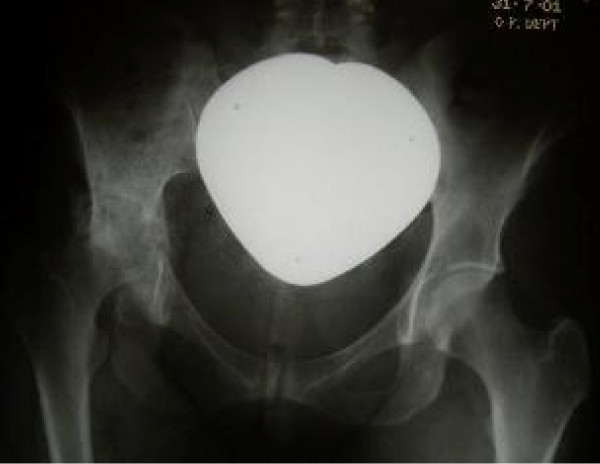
Radiograph showing involvement of Right ilium and hip by the Hydatid disease.
